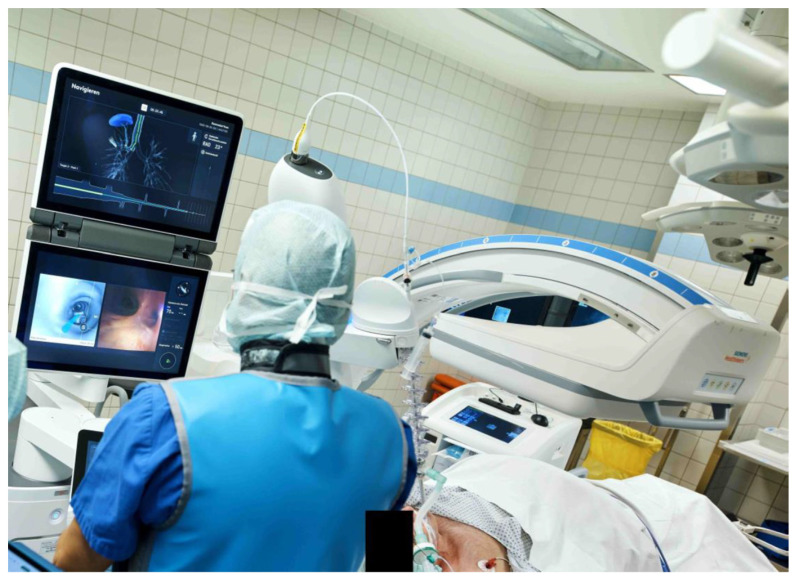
Operating room setup with ION ssRAB and CBCT.
